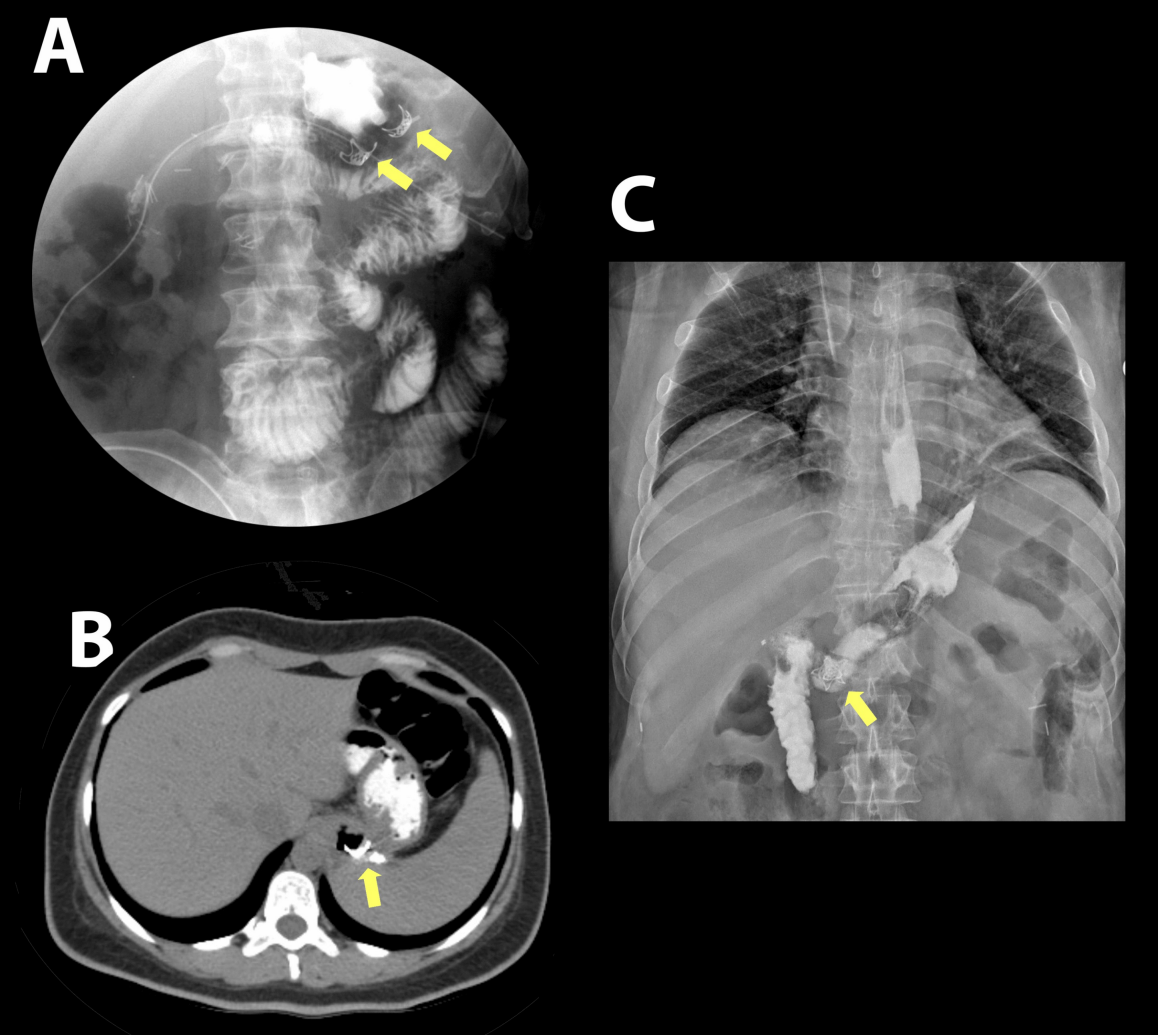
Follow-up imaging (A) Fluoroscopic image showing two OTSCs with Gastrografin, demonstrating no leakage. (B) Contrast-enhanced CT scan. (C) Radiograph depicting OTSC in situ. OTSC, over-the-scope clip
